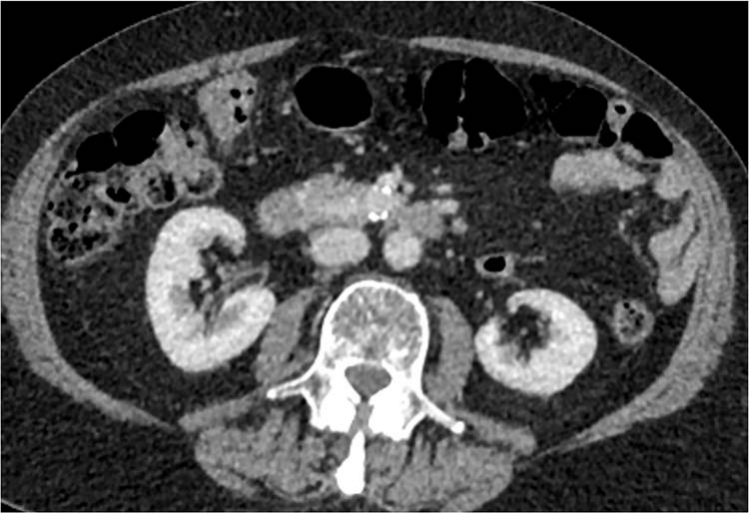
Initial CT: axial view, demonstrating what was initially thought to represent indeterminate pancreatic/duodenal lesion.
